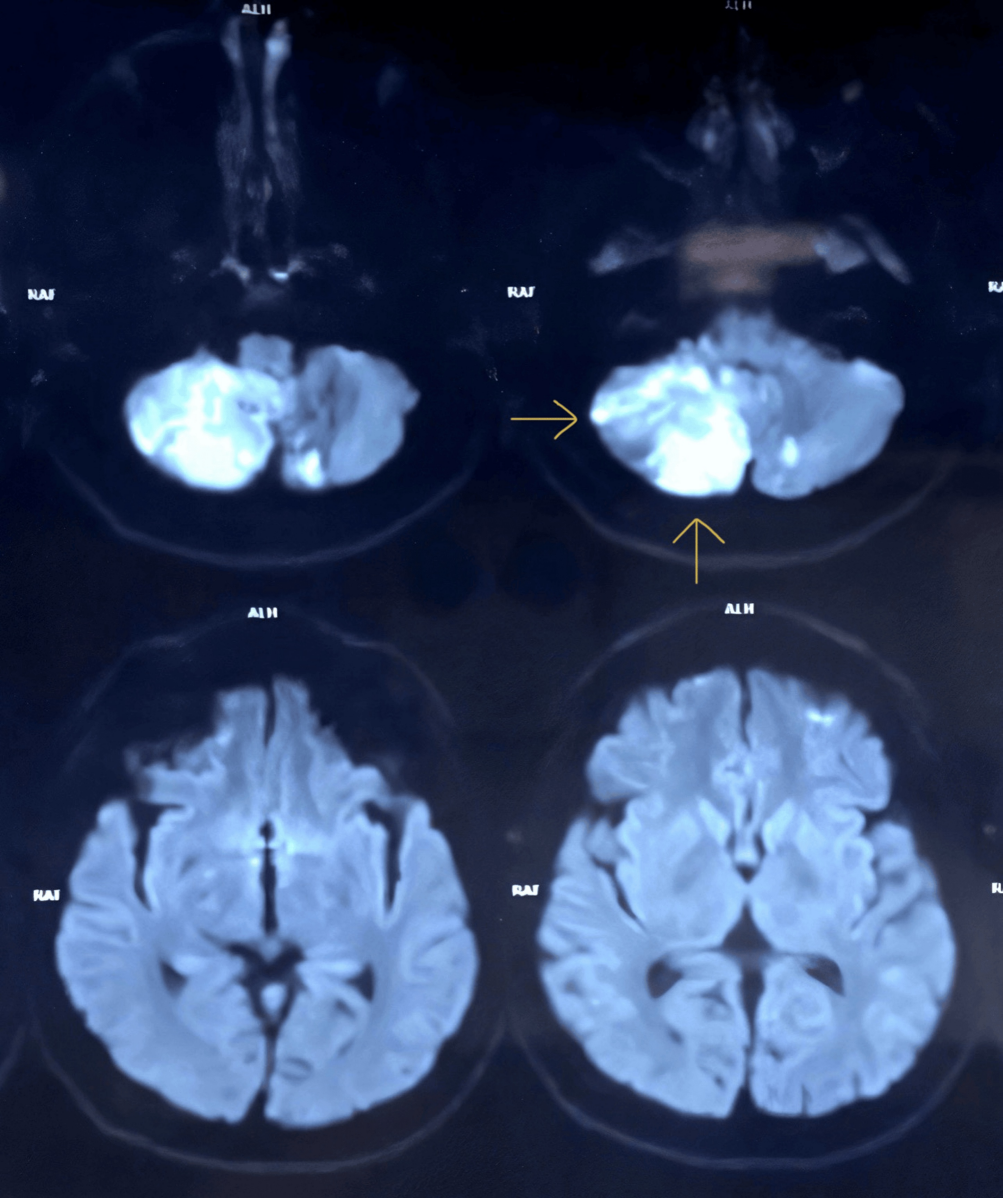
Diffusion-weighted MRI showing acute infarction in the right greater than the left cerebellar hemisphere. Axial diffusion-weighted MRI showing marked hyperintensity in the right cerebellar hemisphere with a small contralateral focus in the left (arrows), consistent with acute ischaemic infarction. MRI: magnetic resonance imaging
